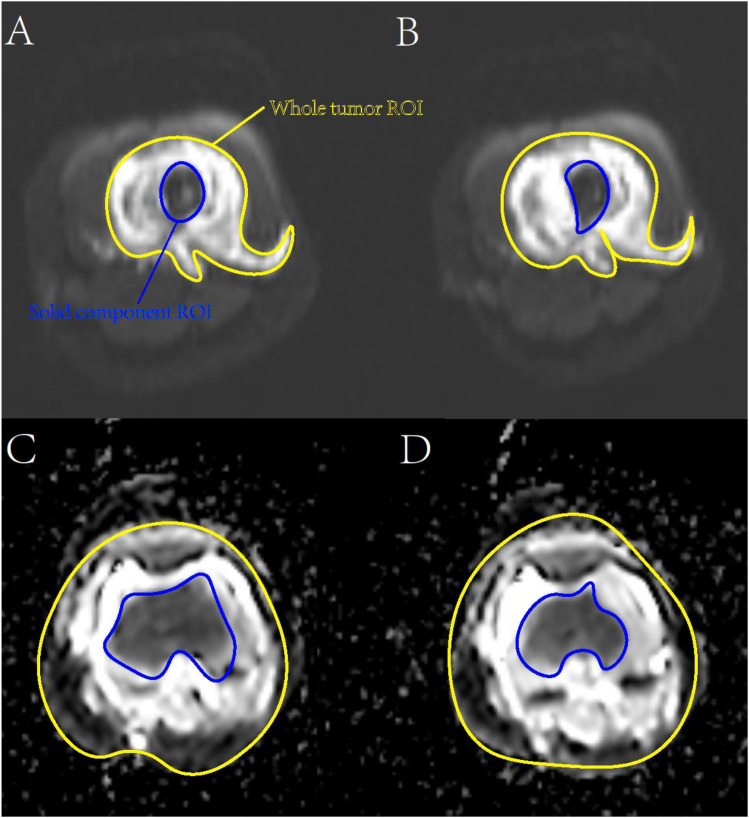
DWI map and ADC map. (A) DWI map of good prognosis; (B) DWI map of poor prognosis; (C) ADC map of good prognosis; (D) ADC map of poor prognosis.
